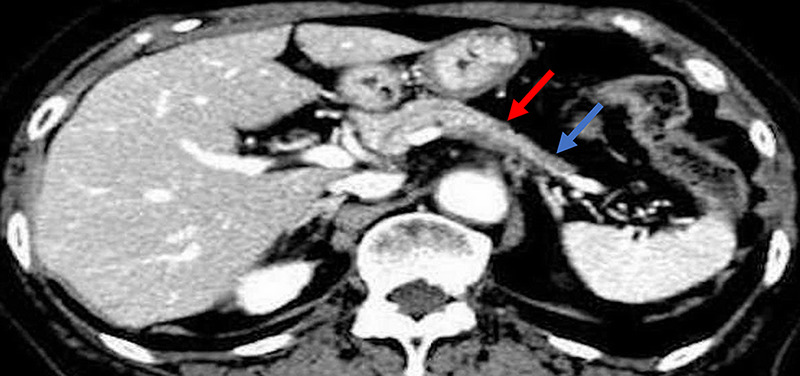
Contrast-enhanced computed tomography showed an 11-mm nodular lesion (red arrow) in the pancreatic body, which appeared hypodense in the early phase. Furthermore, mild dilation of the main pancreatic duct and atrophy of the pancreatic tail on the caudal side of the nodular lesion were observed. The blue arrow showed mild dilation of the main pancreatic duct and atrophy of the pancreatic tail.
